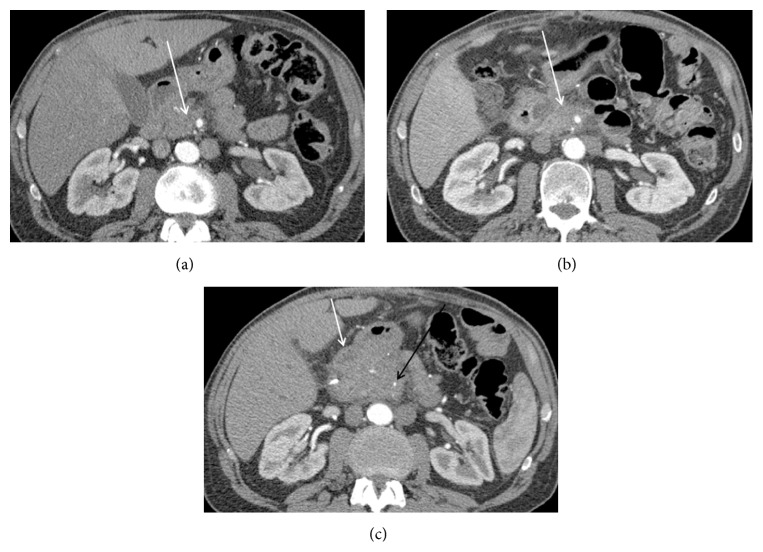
Features worrisome for recurrence. (a) Pre-op CT, a soft tissue mass (white arrow) is seen surrounding the SMA; (b) immediately post-op, hazy amorphous soft tissue (white arrow) is seen surrounding the SMA. There is some enhancement of the ablation bed; (c) 2 months post-op, there is new narrowing of the SMA (black arrow) with enlarging soft tissue at the postablation zone. Its anterior borders cannot be separated from adjacent bowel (white arrow). Increased volume and new narrowing of a vessel are suggestive of recurrence.
